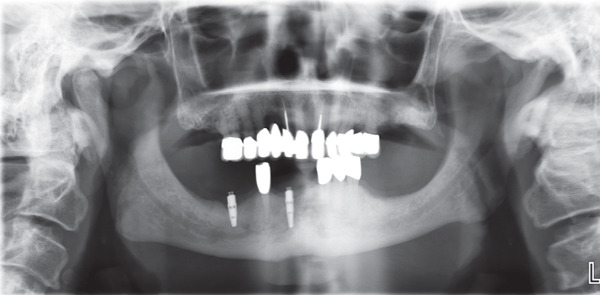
Panoramic radiograph after completion of dental treatment.
